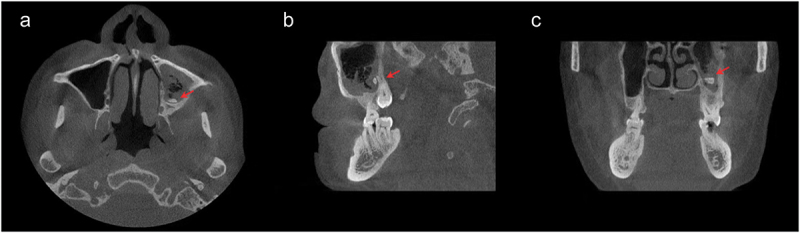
CBCT (A–C) Revealed that a high-density image of a suspected broken tooth in the left maxillary sinus.
